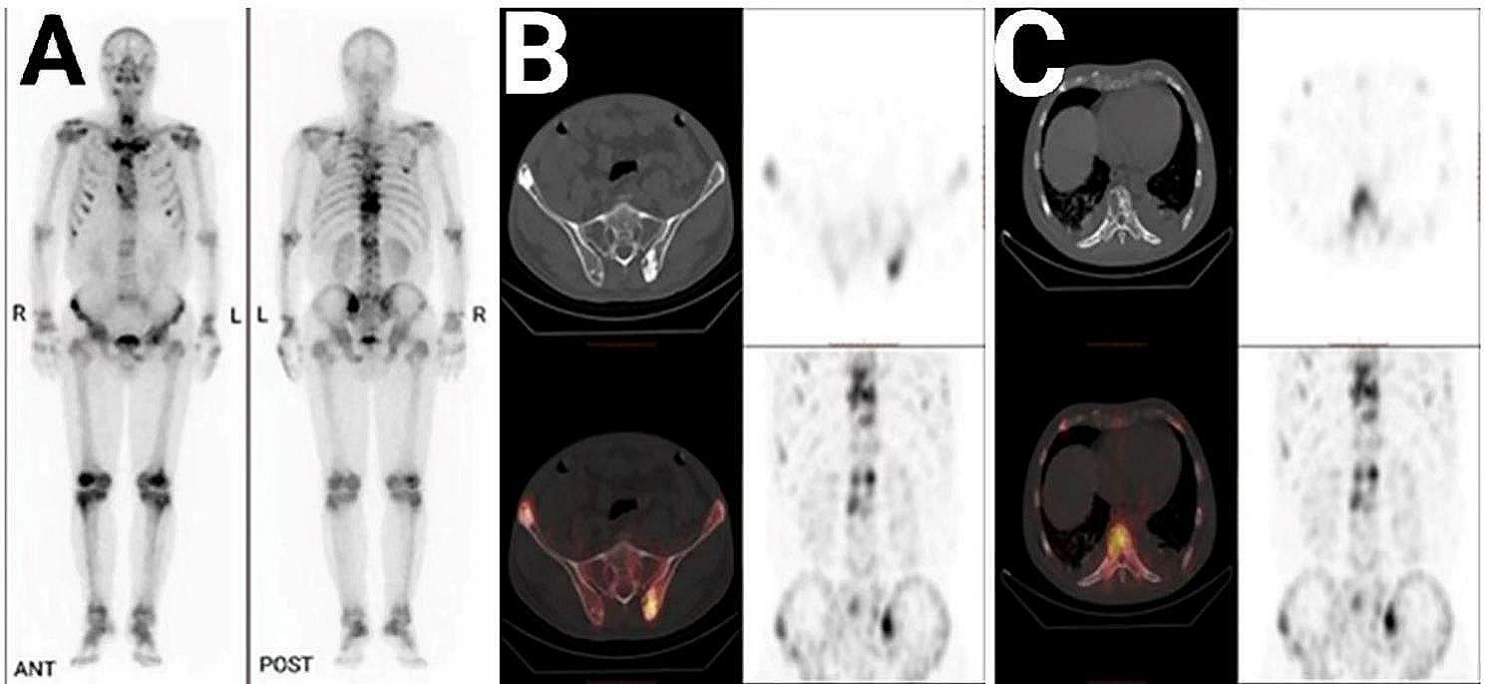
The results of bone scintigraphy with [99mTc]-MDP SPECT/CT. (A) Three hours after intravenous injection of the imaging agent, bone scintigraphy revealed enhanced bone metabolism in multiple parts of the body. The local SPECT/CT fusion images showed the coexistence of osteogenic and osteolytic bone destruction of (B) bilateral iliac crest, and (C) vertebrae and ribs. Abbreviations: MDP SPECT/CT: methylene-diphosphonate Single Photon Emission Computed Tomography/Computed Tomography; R: right; L: left; ANT: anterior; POST: posterior
